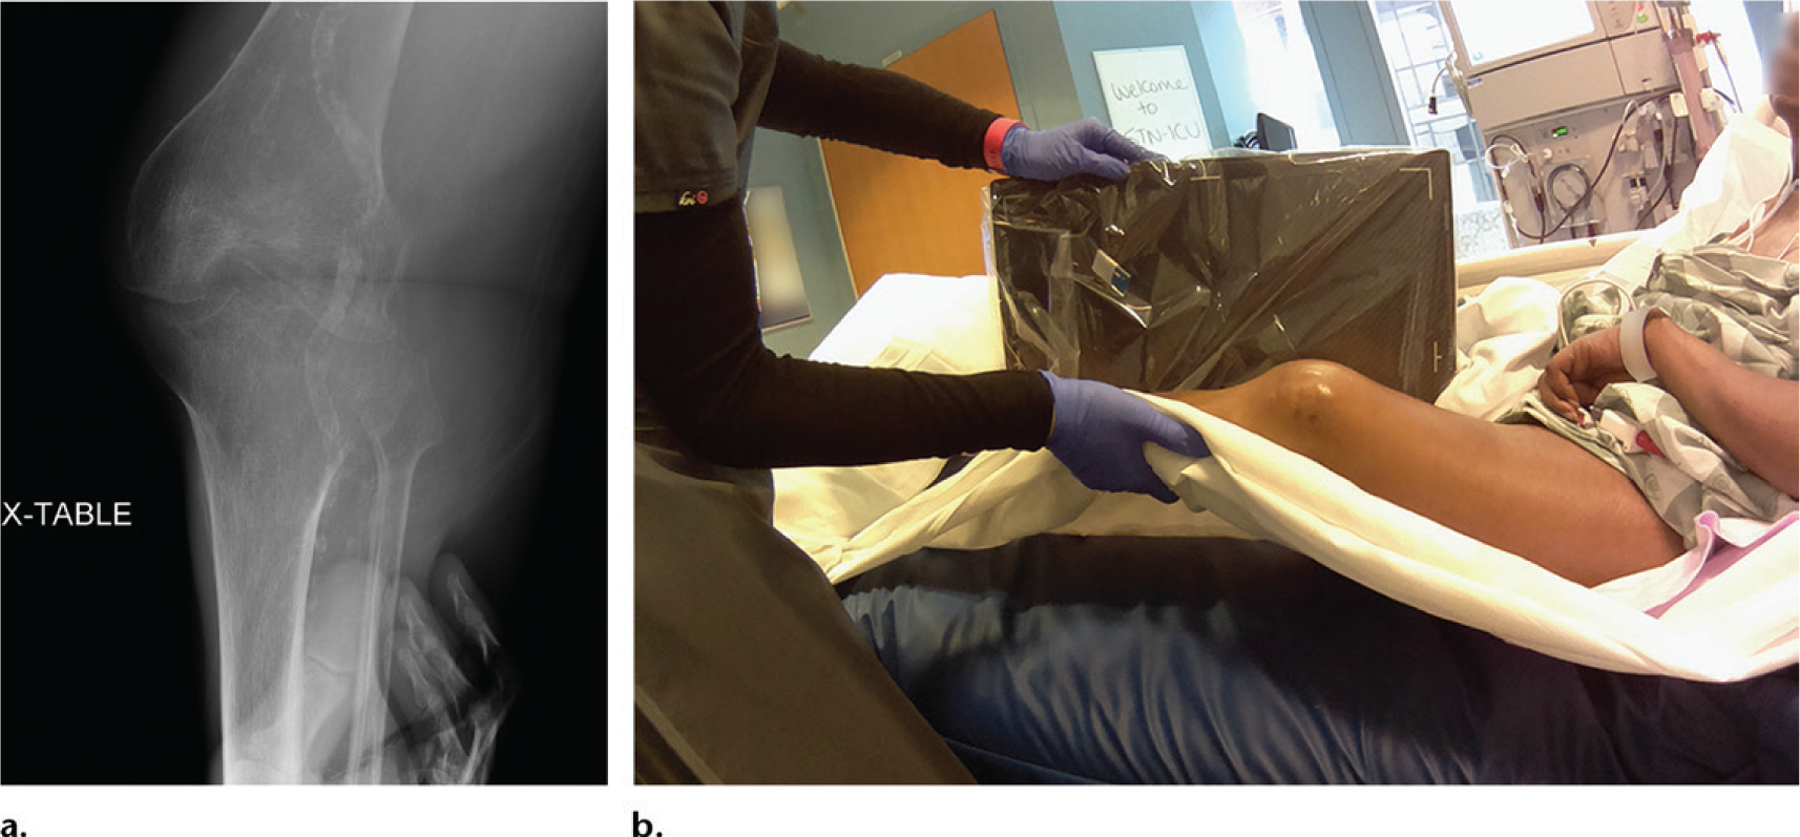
(a) Cross-table lateral radiograph of the knee obtained in a suboptimal patient position. Laterality information was not provided on the radiograph. (b) Photograph shows the physical challenges the technologist faced in properly obtaining this view in this patient, who could not cooperate. The photograph documents the medicolegal explanation for the suboptimal examination, while making it clear that the left knee was being imaged.
